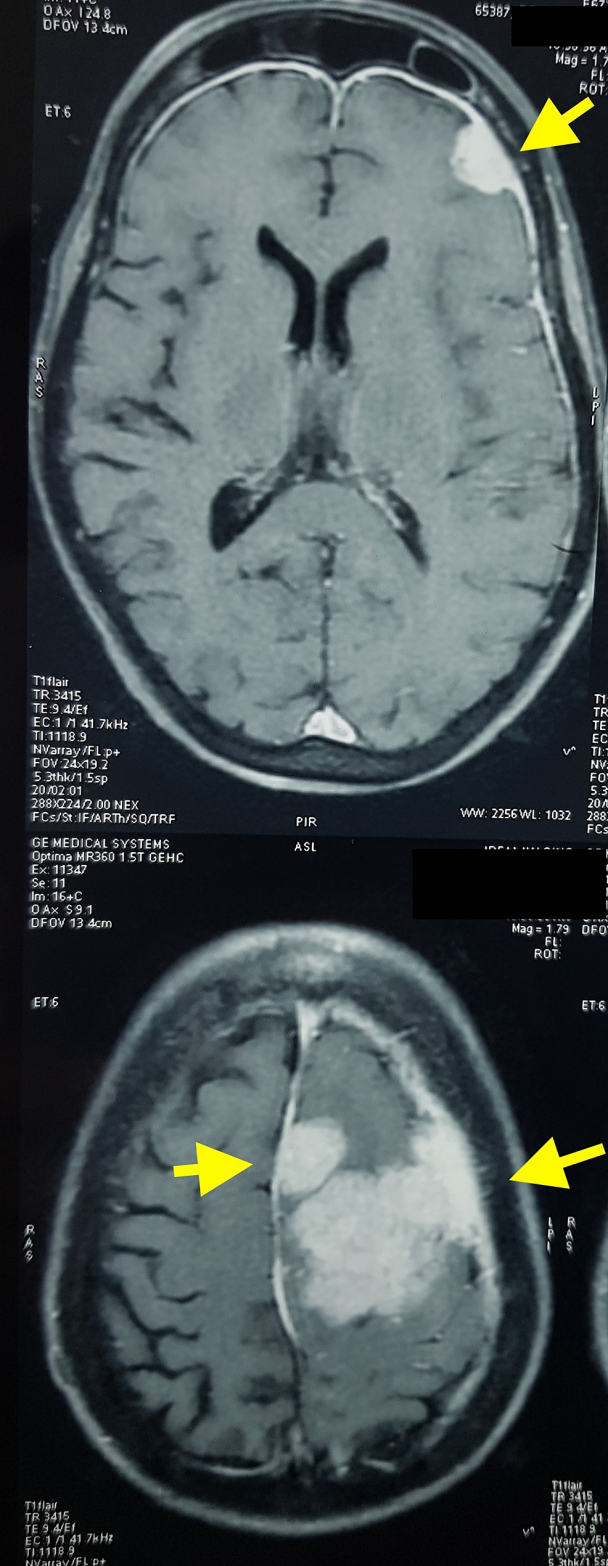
Pre-operative MRI axial images showing multiple meningiomas.
